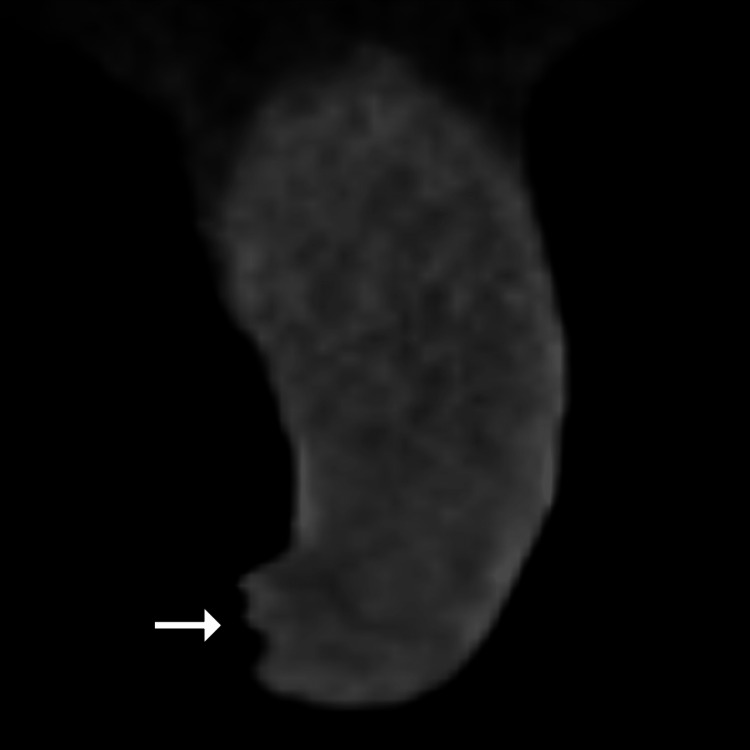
Coronal unenhanced CT in a different patient. An unenhanced coronal CT image in a different 84-year-old man without paraphimosis shows the normal relationship of the prepuce with respect to the glans penis (arrow).
